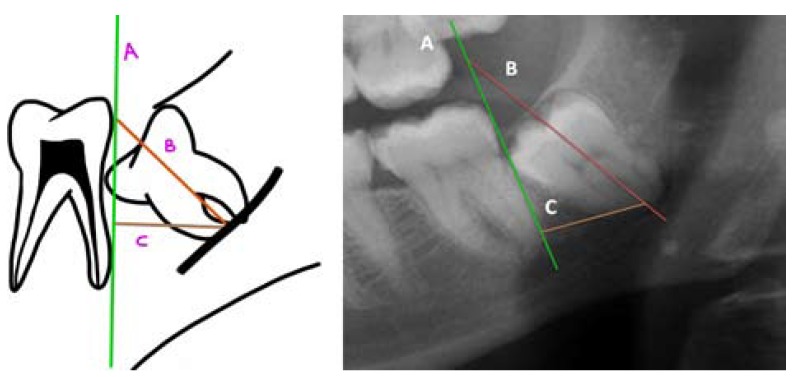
a) Calculation of the root migration according to the technique proposed by Goto *et al*. (18). Lines A (tangential to the second distal molar), B (center of third molar) and C (perpendicular to A and which goes in direction of the apical point of the lower third molar) are traced. Compilation prepared by the authors, based on the technique proposed by Goto *et al*. (18). (b) Example in the form of a radiographic image.
